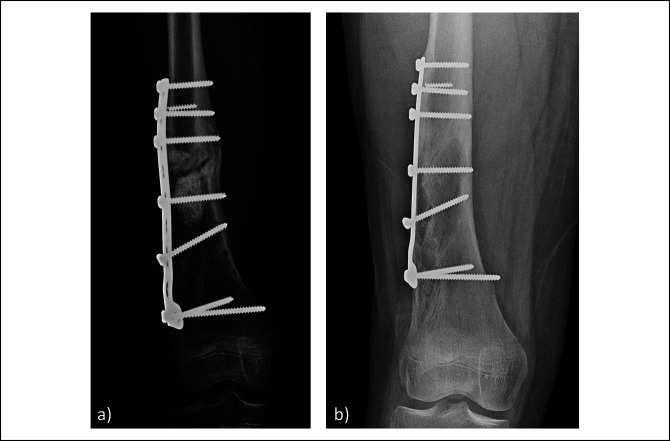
A, AP radiograph of the right distal femur of a 12-year-old boy demonstrating revision surgical fixation of a pathologic fracture. B, AP radiograph of the right distal femur 2 years later showing the cutback phenomenon of the most distal screw. Significant growth of the distal femur has occurred compared with the previous radiograph. The most distal screw that was previously in the metaphysis is now metadiaphyseal and more prominent.
